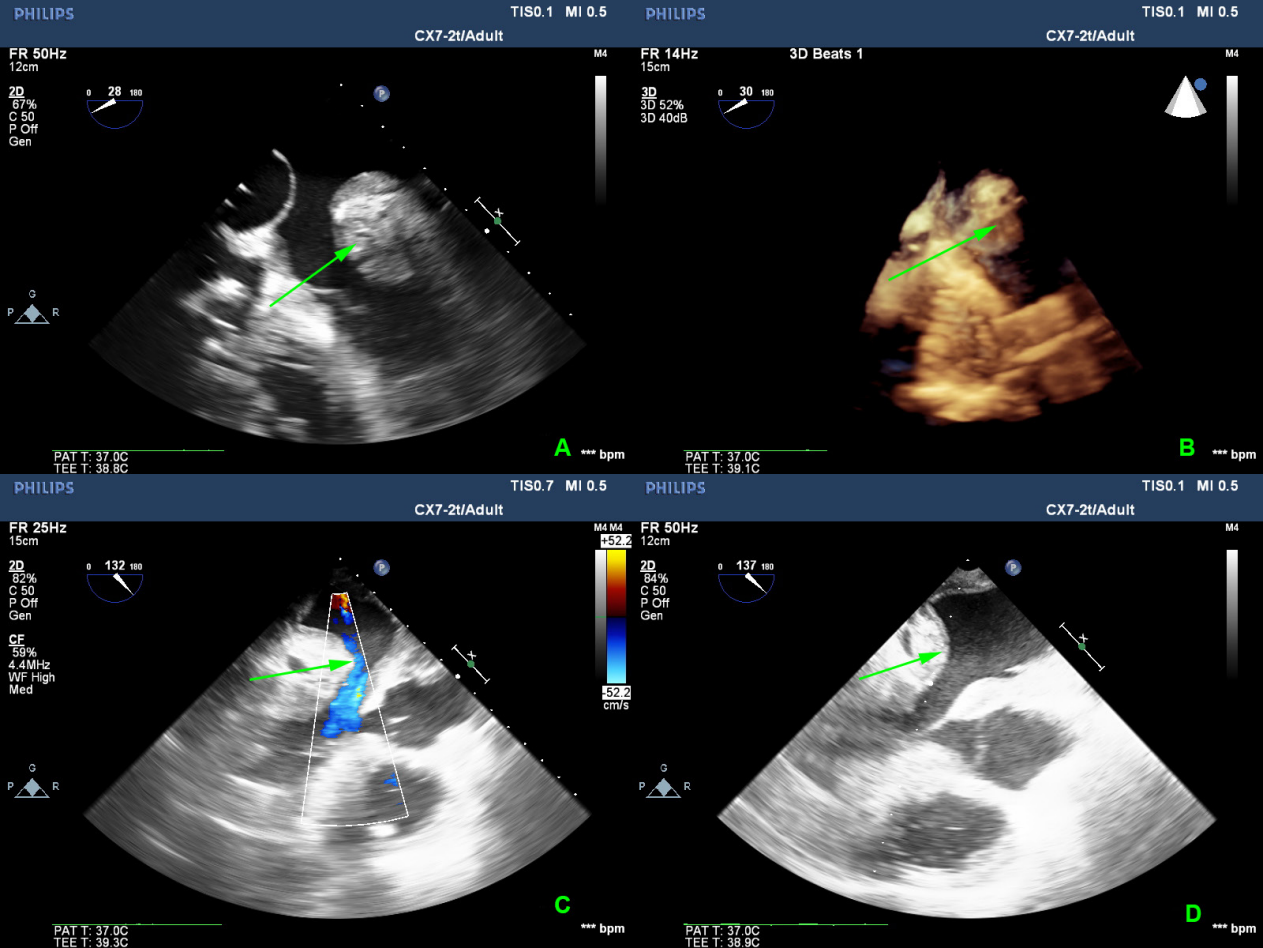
(A) Intraoperative echocardiogram showing left atrial dissection; (B) 3D
